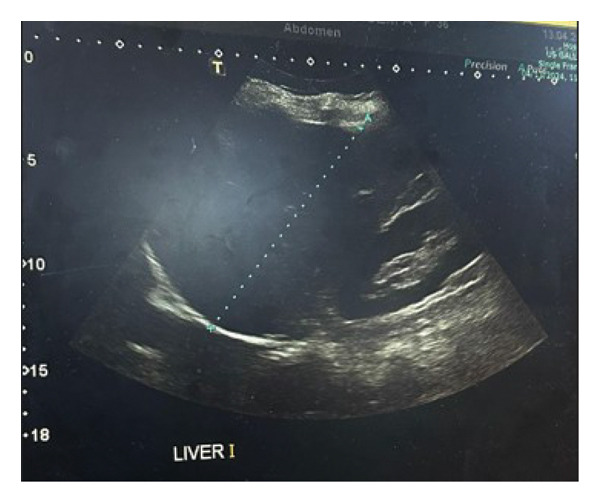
Normal liver ultrasound that excludes any liver metastasis.
